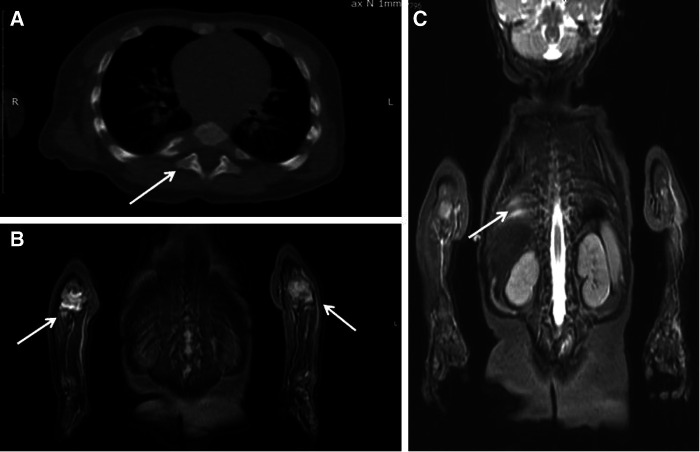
Patient 1: (A) chest CT: the destruction of the head of the 7th right rib (arrow). (B,C) Whole-body MRI (COR STIR sequence) Bone marrow edema of the 8th and 9th right ribs (C), synovitis of both elbows (B).
